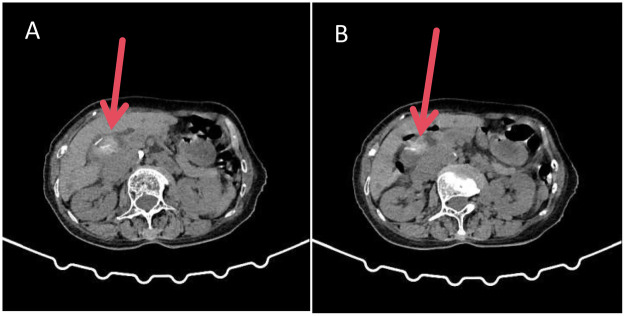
Preoperative upper abdominal CT images. (A) Axial view showing a dilated common bile duct with multiple hyperdense nodules. (B) Coronal view revealing a distinct linear hyperdensity at the center of a dominant stone in the proximal common bile duct (arrow), suggestive of a migrated clip.
